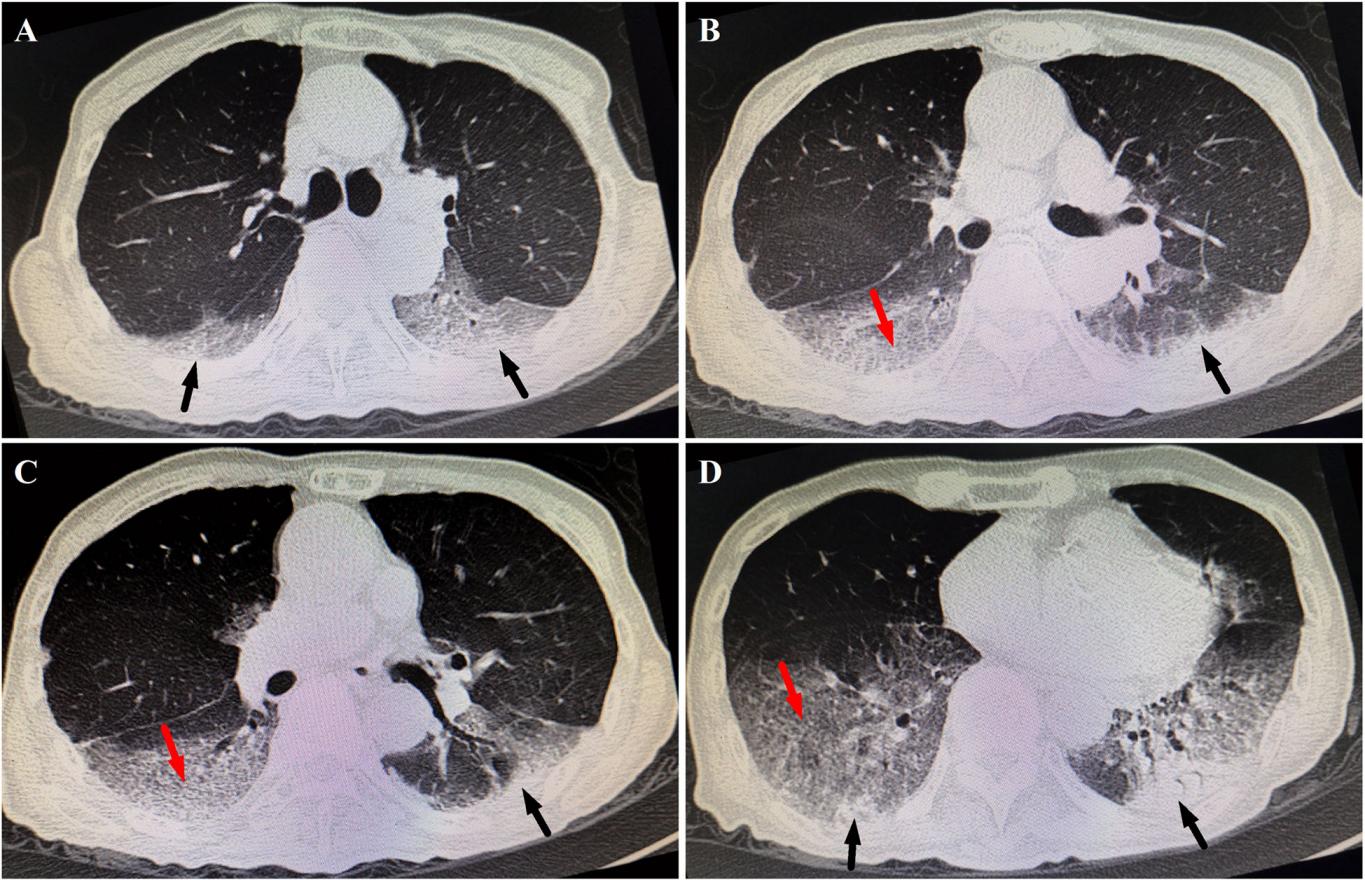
Selected images from chest computed tomography scans at different levels on hospital day 5. Subpleural multiple consolidations (black arrows) associated with smooth interlobular and intralobular septal thickening representing crazy paving pattern (red arrows) mainly in lower lobes in both lungs
